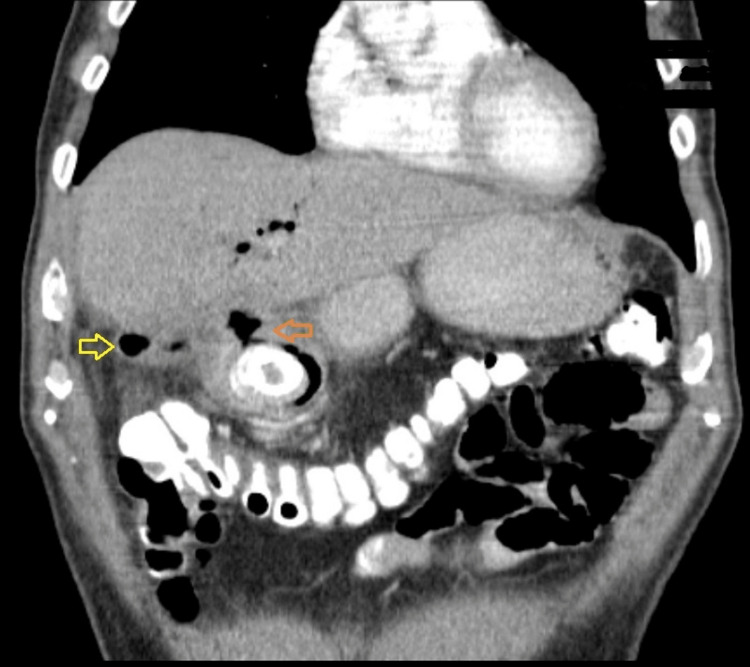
Abdominal CT (coronary reconstruction) Contracted cholecyst (yellow arrow), cholecystogastric fistula (orange arrow), and signs of pneumobilia in the liver parenchyma can be seen.
